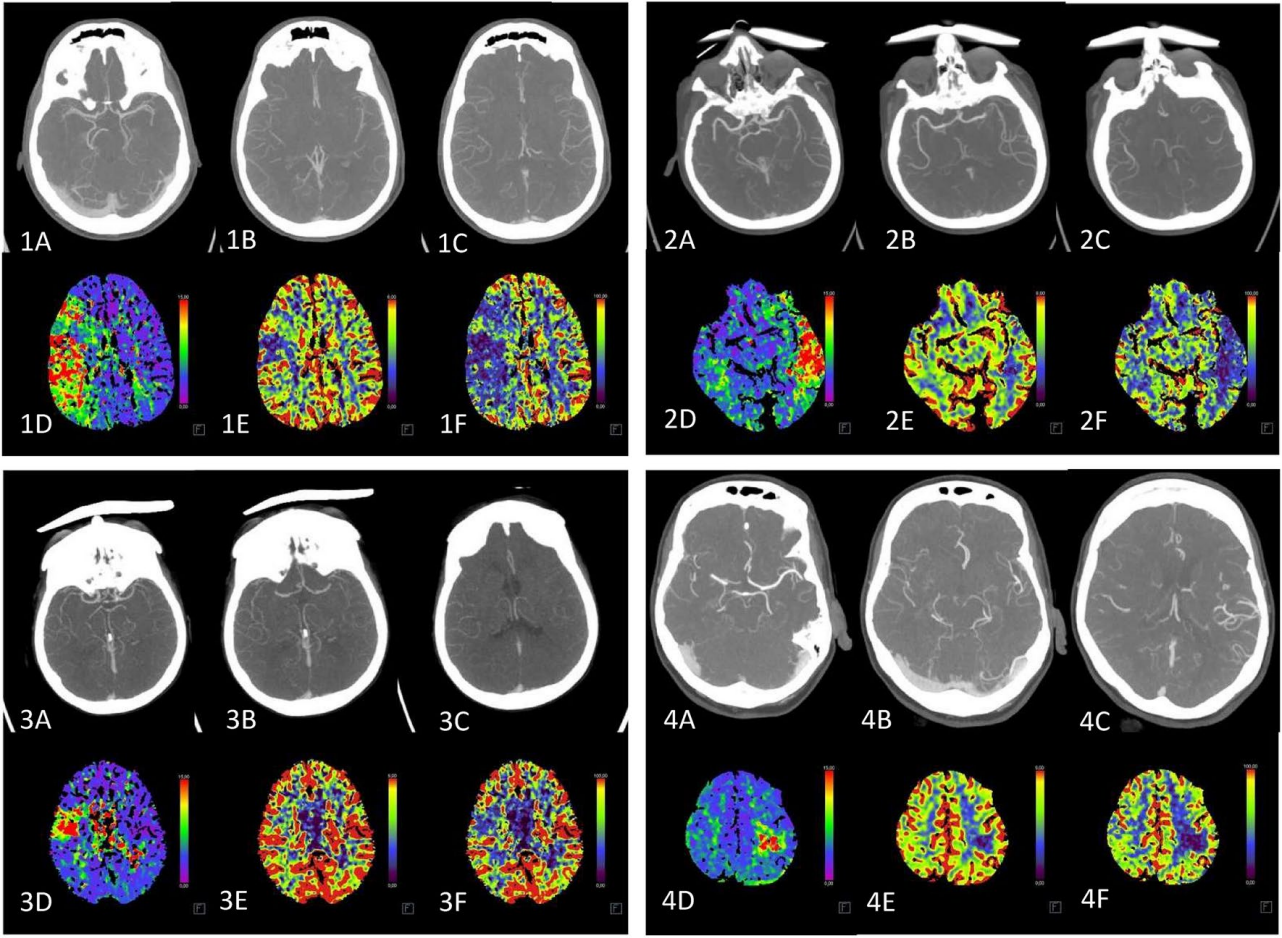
The higher sensitivity of perfusion-Imaging (D-F) compared to CTA (A–C). The CTA is shown representatively as MIP (maximum intensity projection) of the relevant vascular regions. In the CTA, no vessel occlusion is visible although there appears a territorial perfusion deficiency in the TTD (D) as well as a mismatch in CBV (E) and CBF (F).
